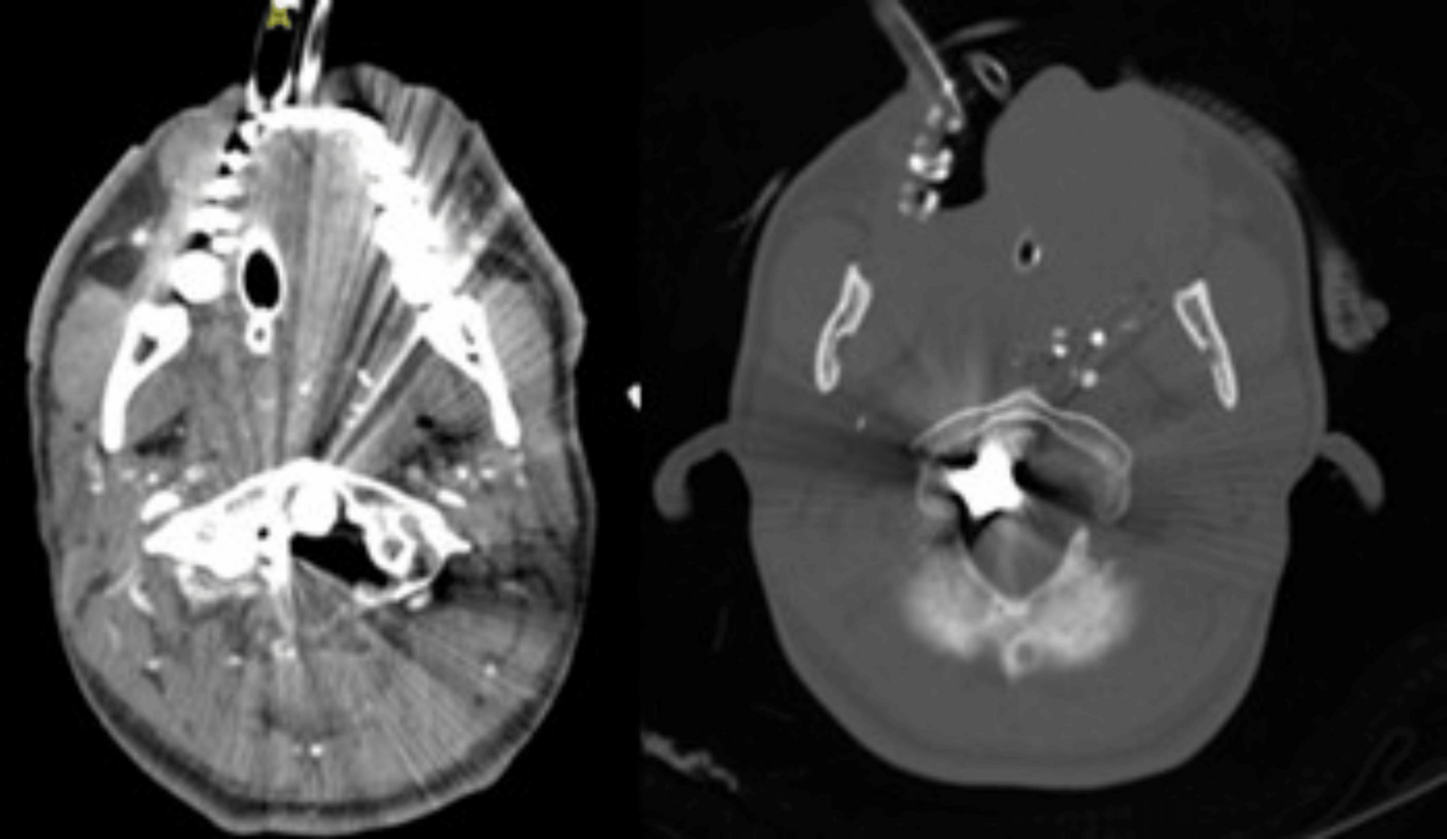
An axial CT scan showed a hyperdense metallic foreign body (bullet) lodged near the lateral aspect of the odontoid process (C2), between the lateral mass of C1 and the odontoid. The image reveals comminuted fractures of the anterior arch of C1, with partial disruption of the cortical structure of the odontoid process. There is significant narrowing of the spinal canal due to the retropulsion of bone fragments, resulting in ventral compression of the cervicomedullary junction. Metallic artifacts from the bullet obscure finer details of adjacent structures, but surrounding fractures and soft tissue damage are clearly delineated. CT, computed tomography
